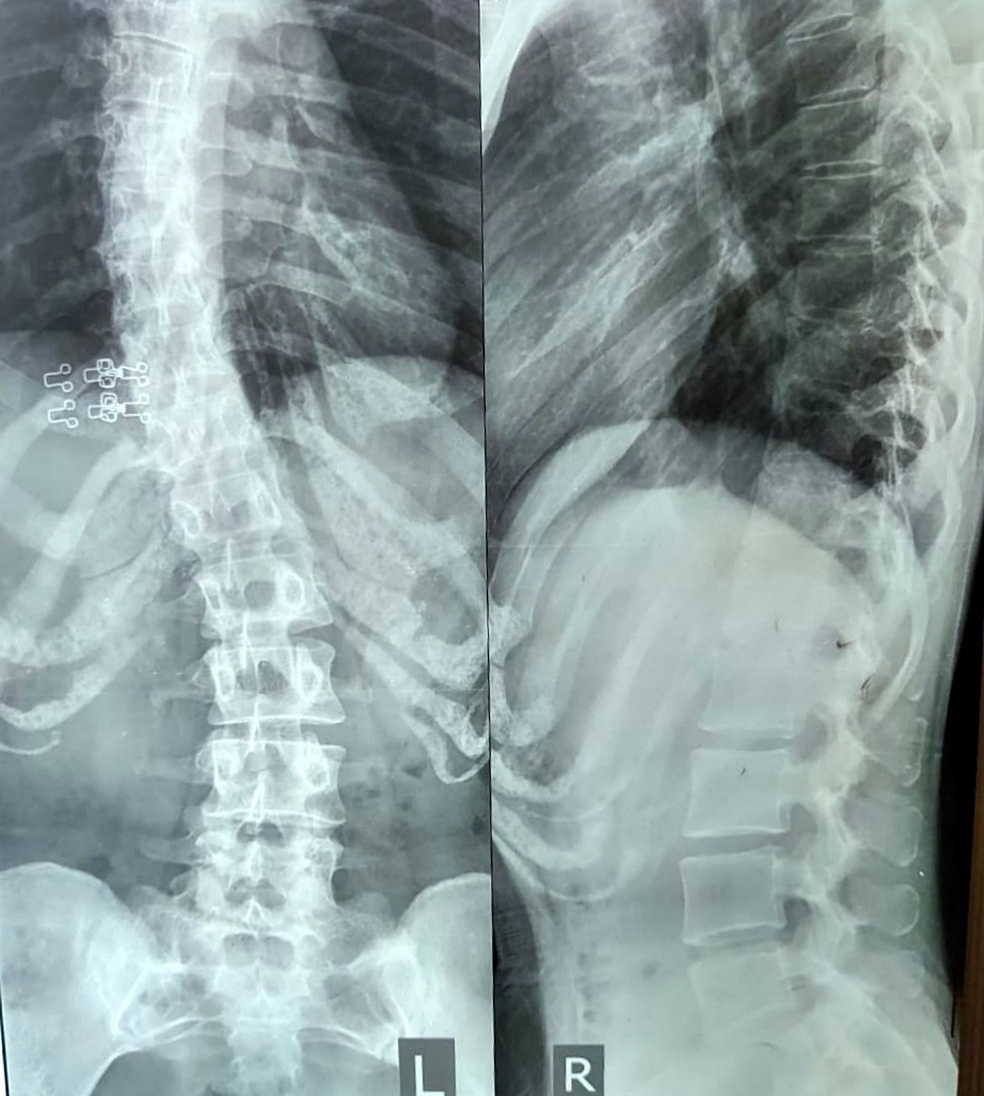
X-ray of the spine (anterior-posterior view and lateral view) The scoliotic curve is noted with tilting of the spinous process and reduced intervertebral space.
